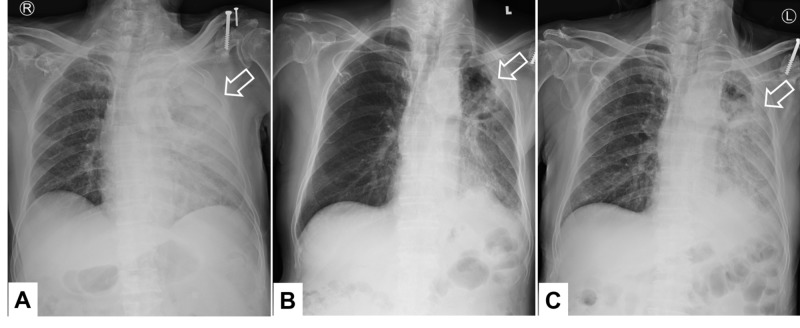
Chest X-ray films along the clinical course: A. March 19, 2019, B. April 30, 2019, C. May 13, 2019 Arrows: Targets of interest
